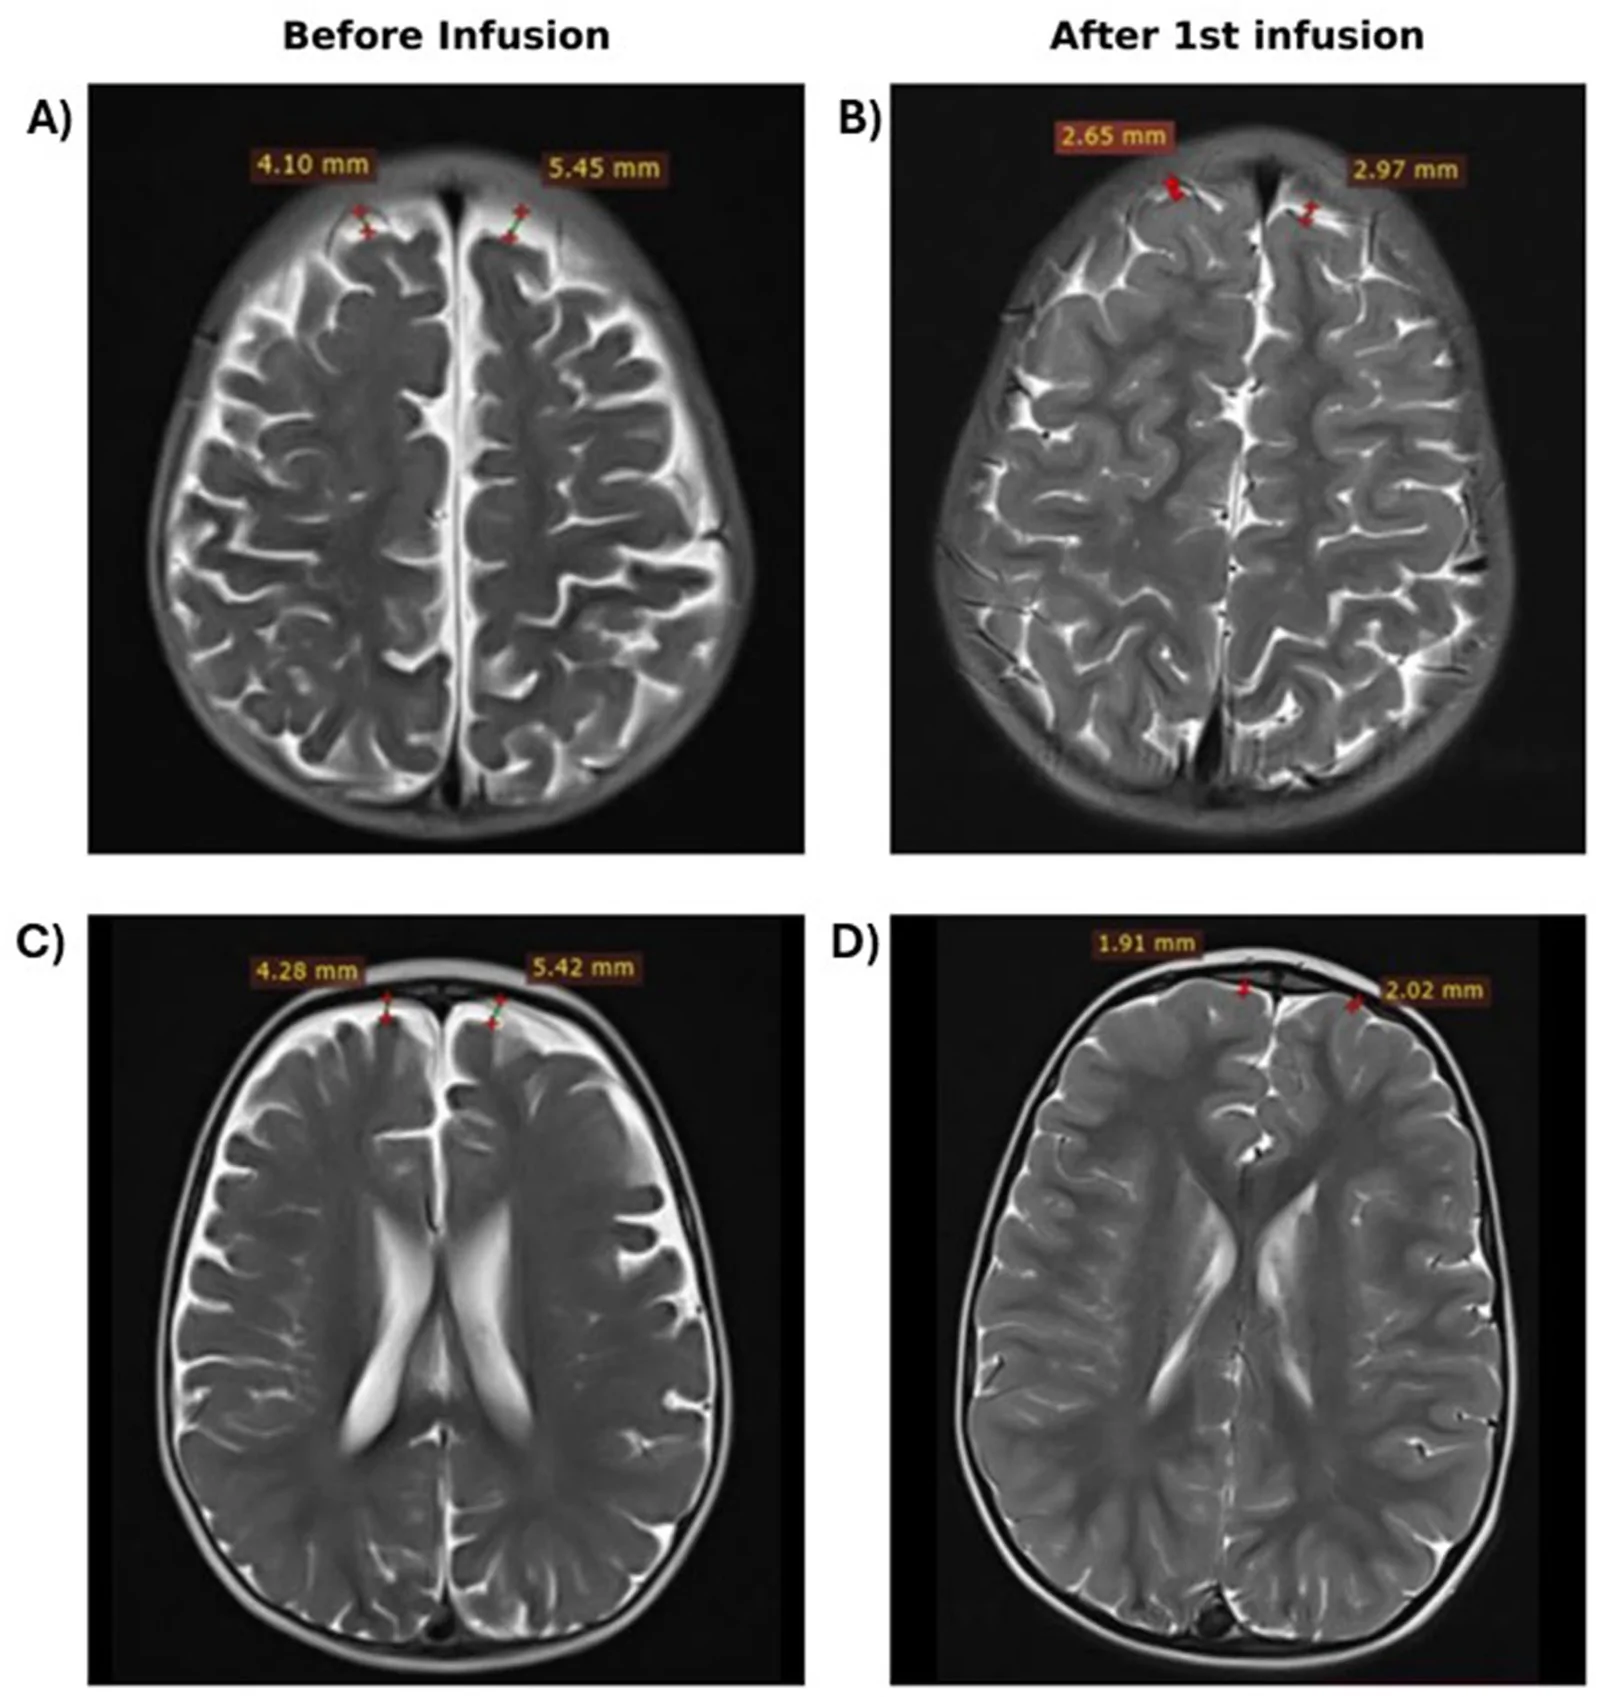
Axial T2-weighted magnetic resonance imaging (MRI) patterns in cerebral palsy following hypoxic–ischemic injury after cardiac arrest CP changes after cell therapy. (A, C) Axial T2W images obtained before cell infusion demonstrated widening of the superficial subarachnoid space (arrows). (B, D) This space was visibly reduced 21 months after the initial cell infusion.
